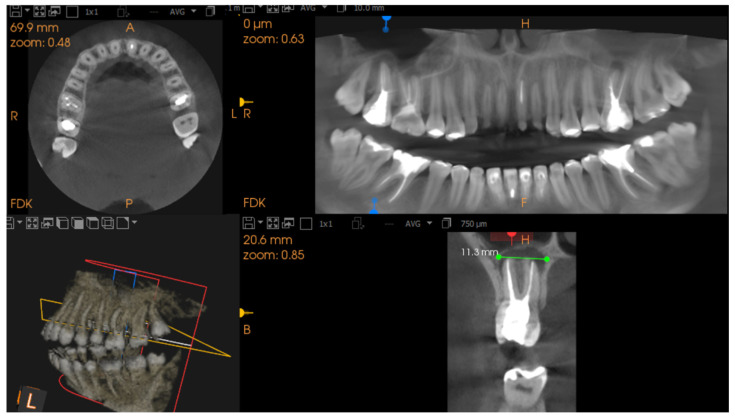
CBCT images demonstrating an extensive periapical lesion of 11.3 mm (marked by double yellow arrows) with poorly defined margins, consistent with a CBCT-PAI score of 5. The multiplanar view allows assessment of lesion dimensions and involvement of surrounding structures.
